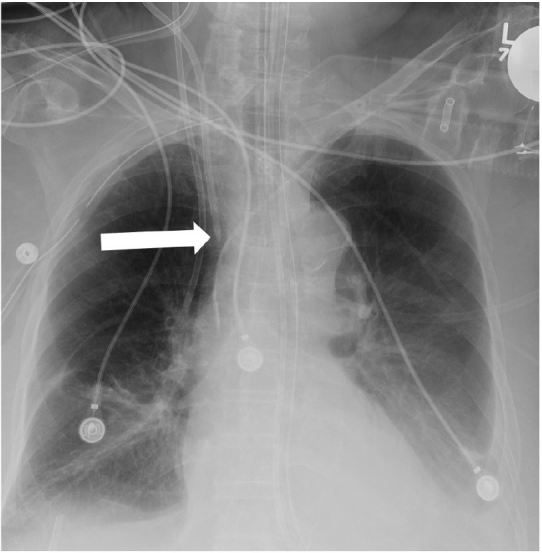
Portable chest radiograph showing mild cardiomegaly and bilateral basal lung opacities. A right internal jugular central venous catheter is shown with its tip apparently located at the superior vena cava. The emergency physician initially read the radiograph as showing the catheter as “adequately positioned.” Poor blood return was observed from the ports during placement. Chest burning pain was present during a normal saline infusion.
